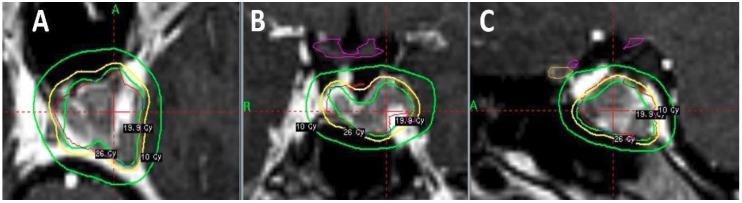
Stereotactic RT in the pediatric setting. This case is that of a 17-year-old female who presents with enlarging residual pituitary adenoma. The patient was initially treated one year prior with subtotal trans-nasal endoscopic resection of a non-secreting pituitary adenoma. On subsequent MRI surveillance, she was noted to have slow enlargement of residual pituitary adenoma. She was therefore recommended for salvage stereotactic RT to this residual disease. She was treated with a single-fraction of stereotactic radiosurgery, prescribing 19.9 Gy to the 50% isodose line. Shown here are representative (A) axial; (B) coronal; and (C) sagittal sections of the plan, with 10 Gy, 19.9 Gy, and 26 Gy isodose lines. Critical structures are appropriately avoided using this highly-conformal single-treatment technique, including optic chiasm (purple contour) and left optic nerve (orange contour).
